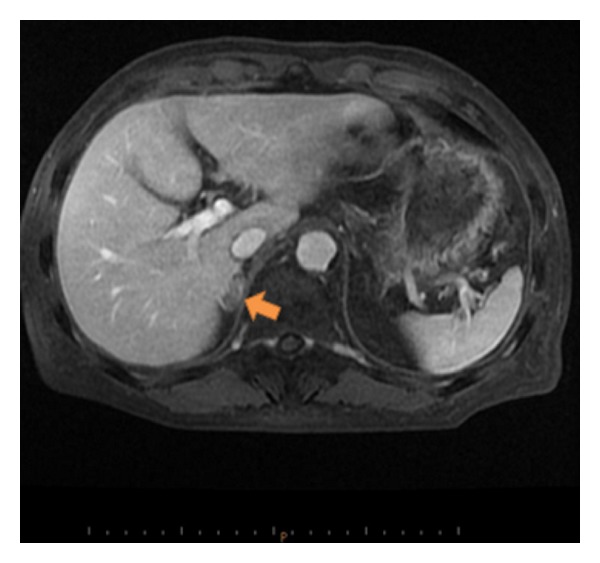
Surveillance-enhanced MRI scan showing enlargement of the right adrenal gland seven months after radical cystectomy.
